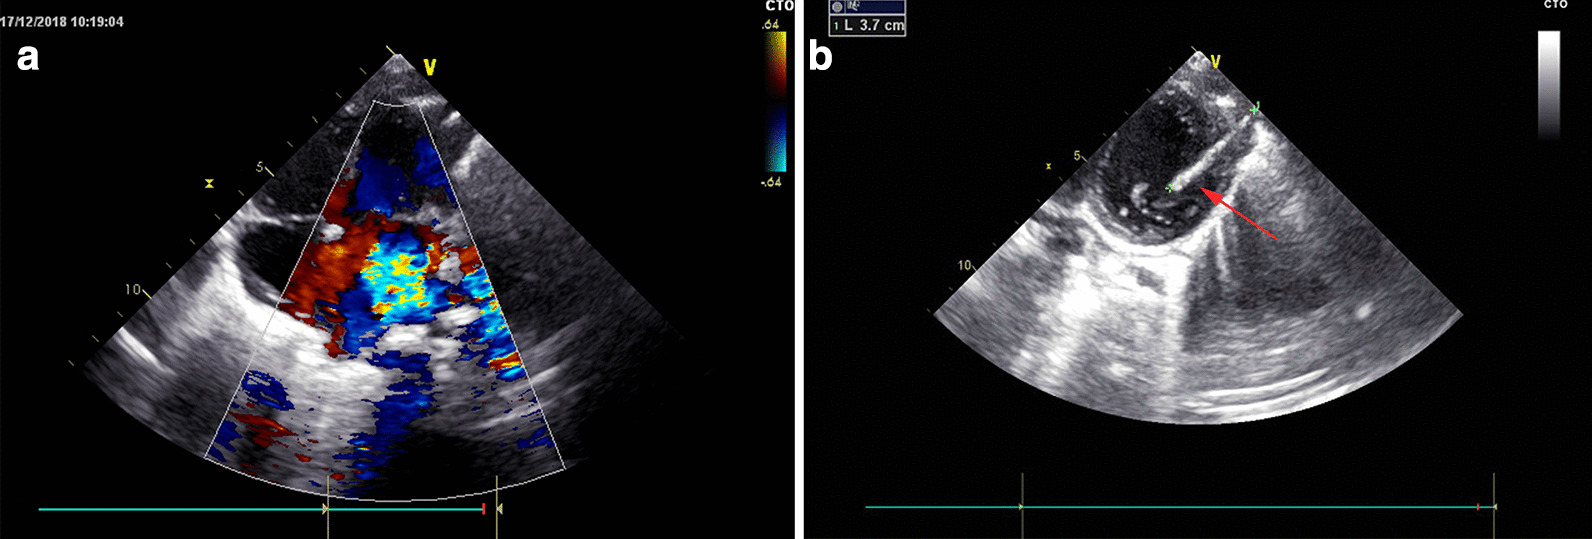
Echocardiography. A Echocardiography showed severe mitral valve regurgitation. B Echocardiography showed the needle in the heart cavity. The arrow indicated the needle
